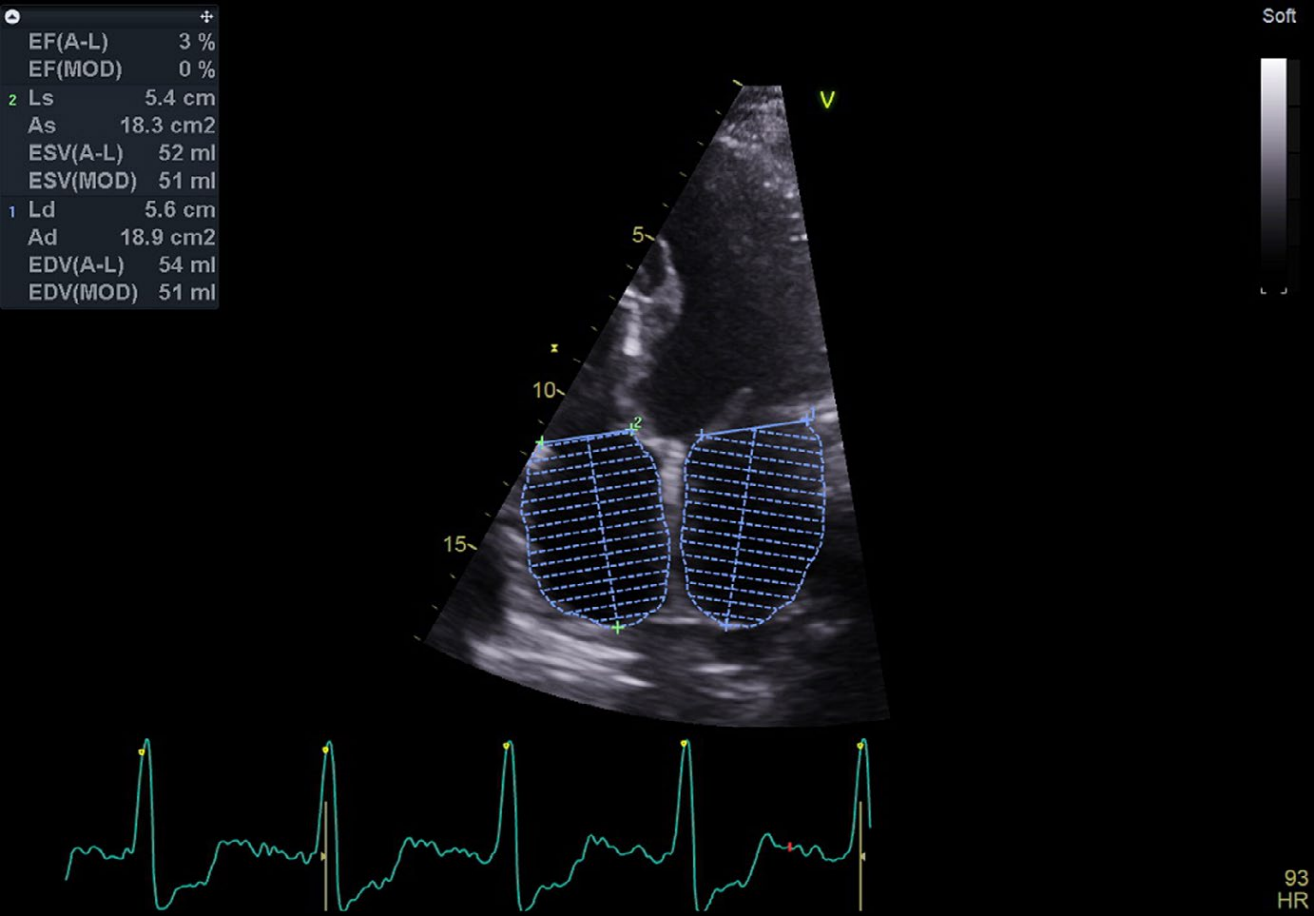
Transthoracic echocardiography after cardiac surgery: Residual mild RA enlargement
